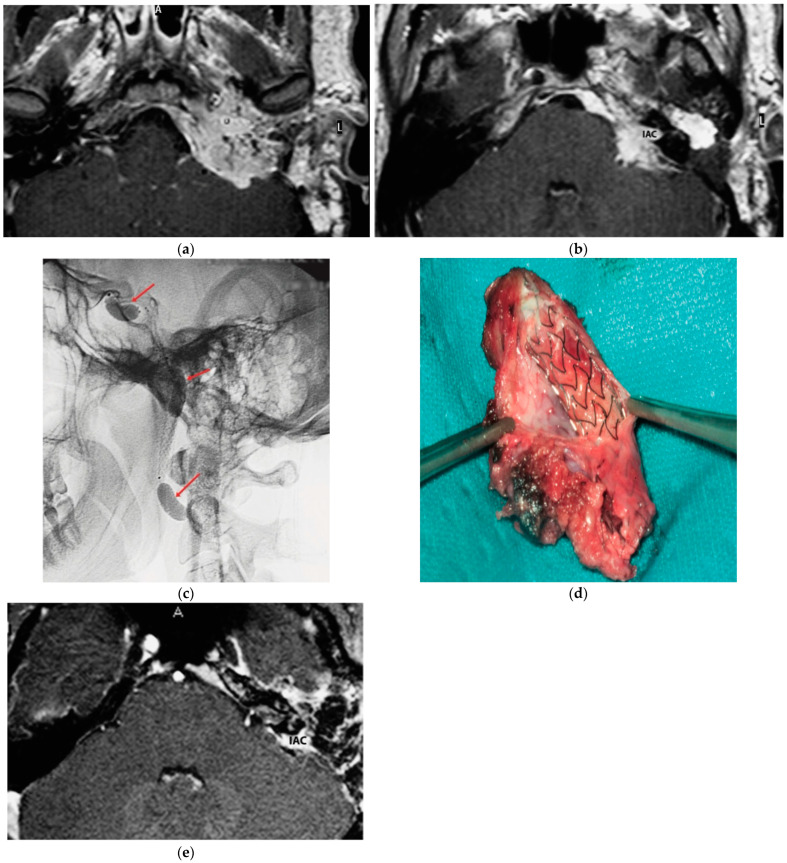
(Case 1) (a) Axial-enhanced T1W MRI, with large intradural tumor extension. (b) Axial-enhanced T1W MRI, with extensive involvement of posterior fossa dura with intradural and IAC involvement. (c) Plain lateral view skull X-ray. Red arrows indicate balloons during permanent occlusion of the IAC. (d) Intra-carotid stent was seen after opening the wall of the carotid artery. (e) Axial-enhanced T1W MRI revealing dural infiltration and the involvement of IAC.
